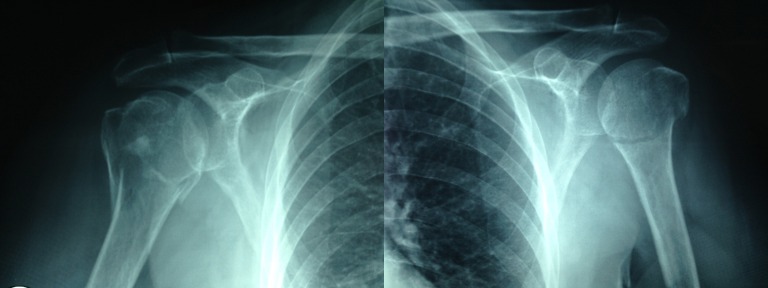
Simple x-ray AP bilateral views, week 1
